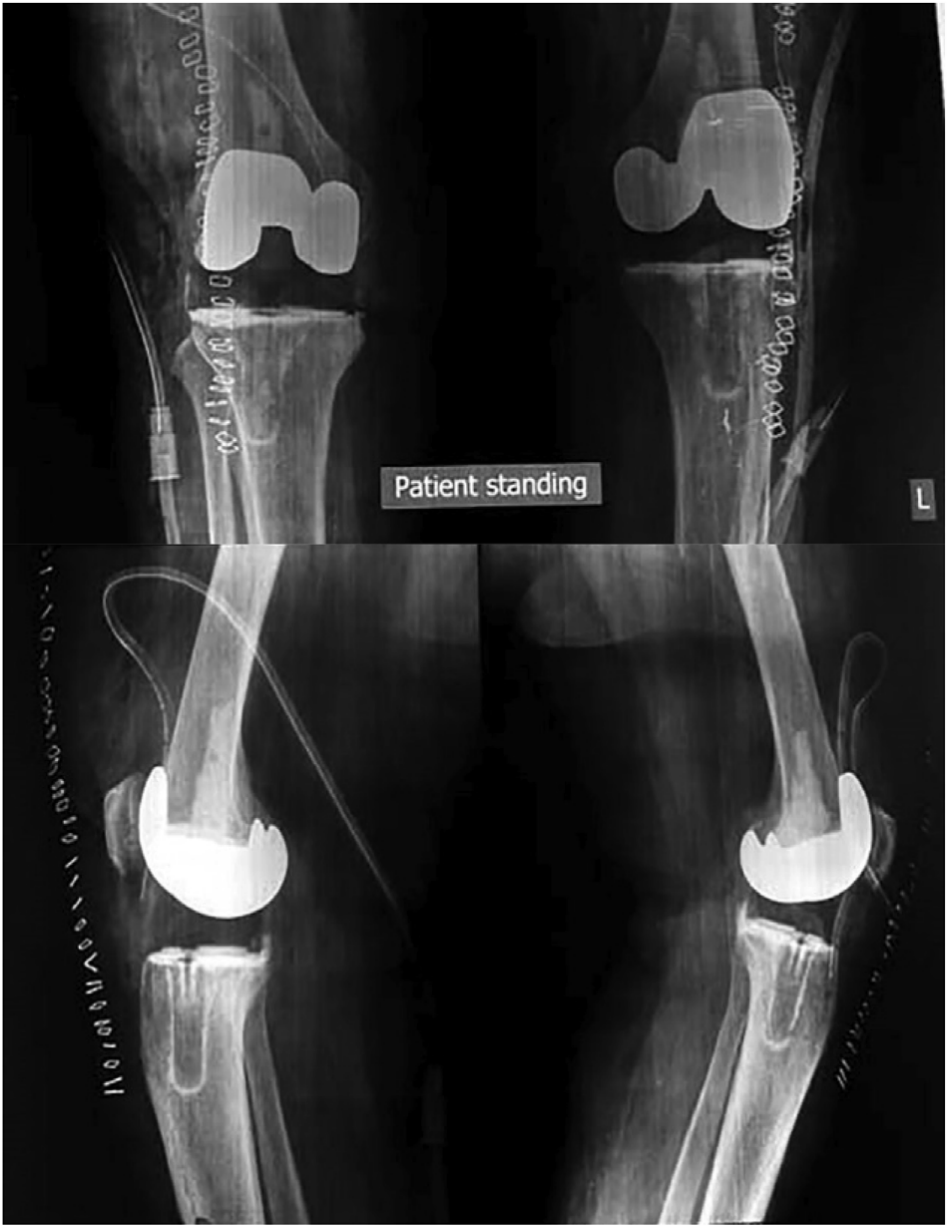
Postoperative x-rays of same patient showing the all poly tibia implant, restoration of joint space and coronal and sagittal alignment.
